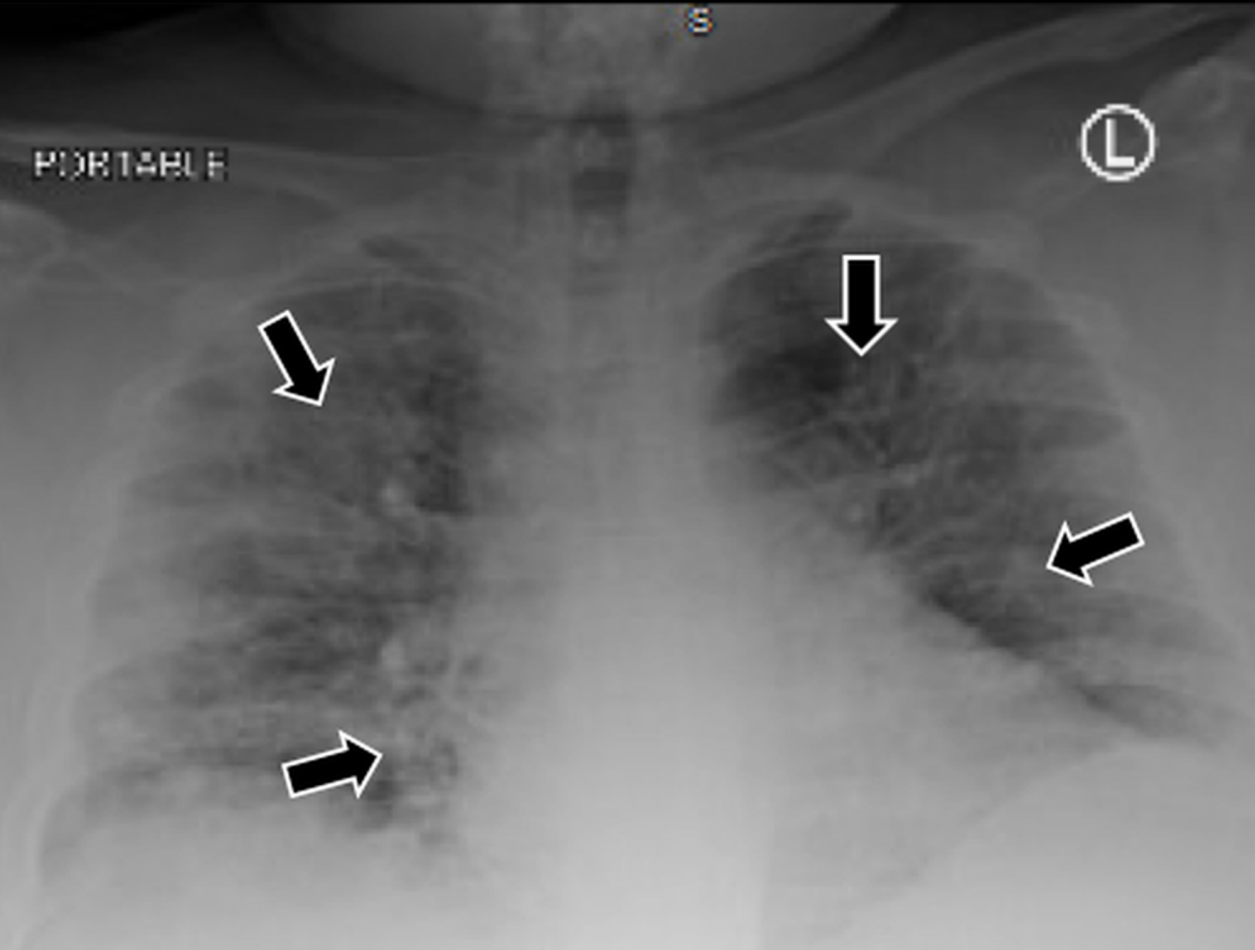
CXR showed bilateral multiple patchy infiltrates CXR: chest X-ray
